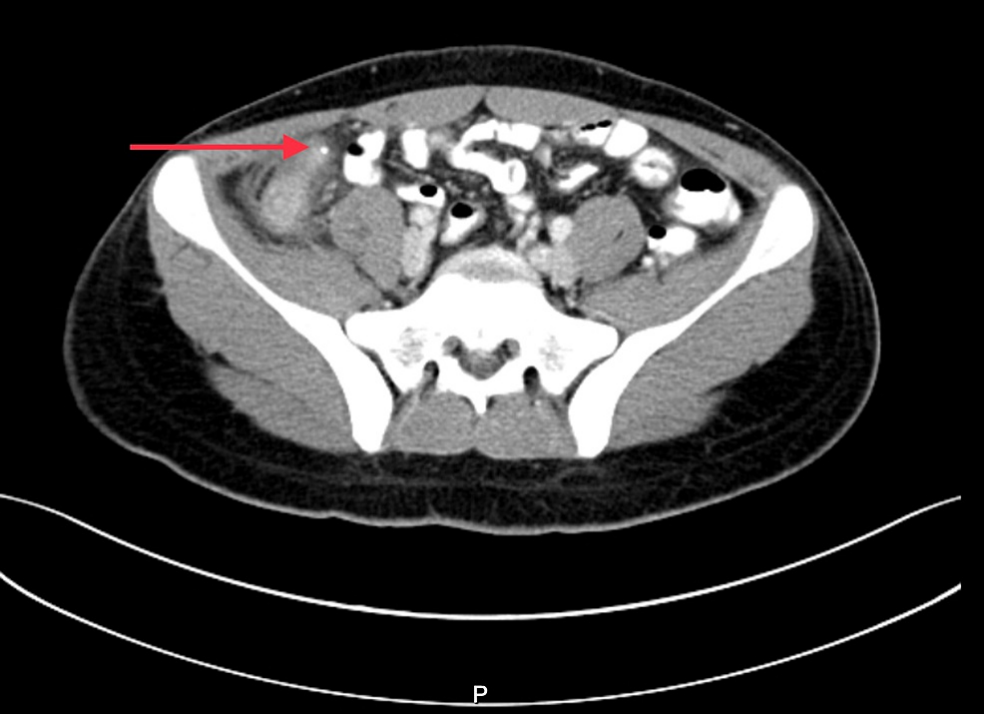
Axial CT Image of the Lower Abdomen With Intravenous and Oral Contrast Shows an inflamed thickened appendix with mucosal enhancement and peri-appendiceal fat stranding. Deep into the appendix is a metallic dot that is incompletely seen on the image
